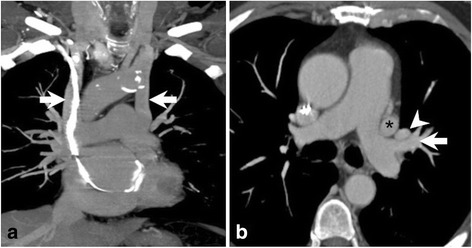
Multiplanar sagittal reconstruction from contrast enhanced CT demonstrate duplication of the superior vena cava (a, arrows), with catheters of a pacemaker coursing into the right sided SVC. Axial image demonstrating the relationship between the left sided SVC (b, asterisk) and the upper pulmonary artery (b, arrow) and upper pulmonary vein (b, arrowhead)
